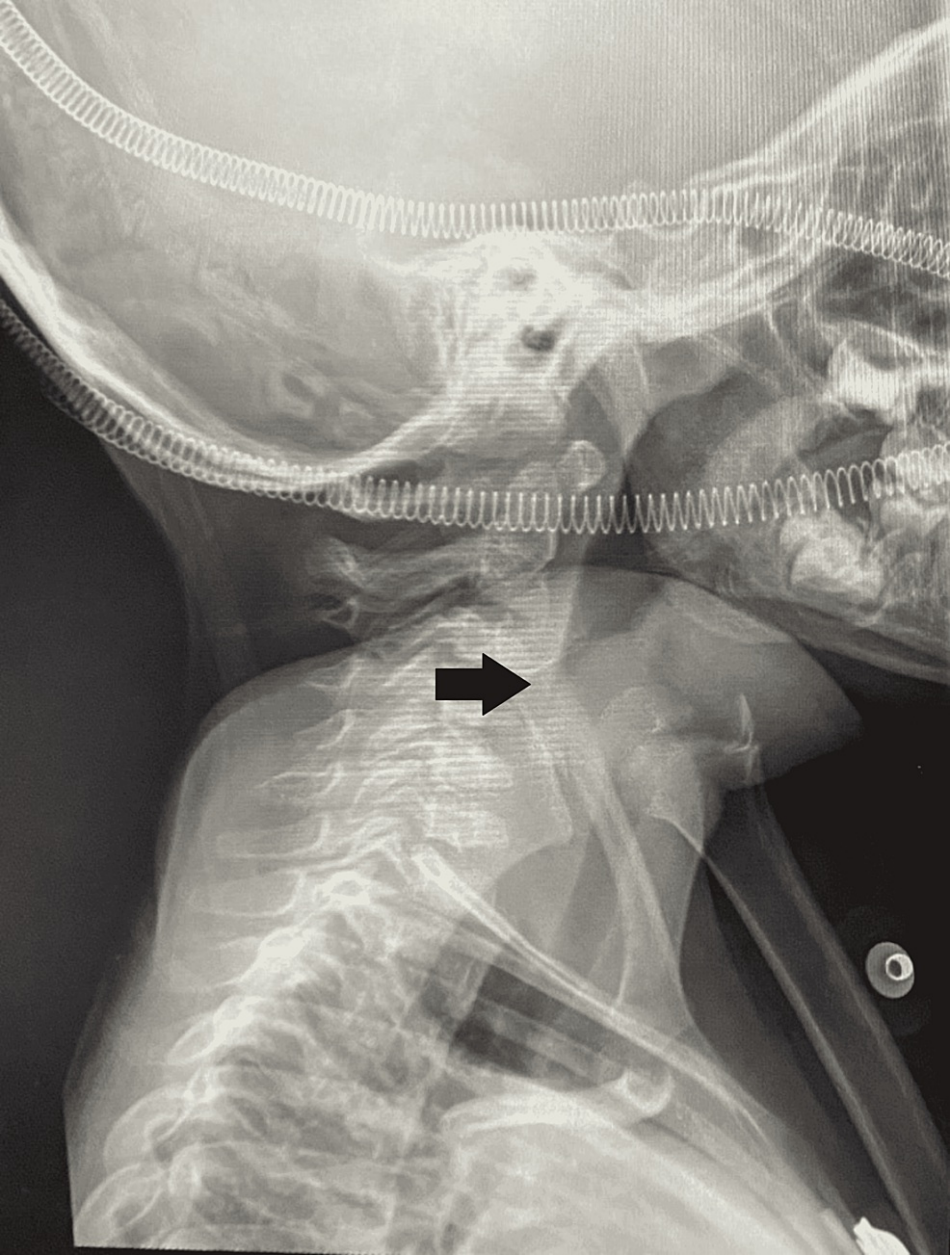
Lateral radiograph of the neck
